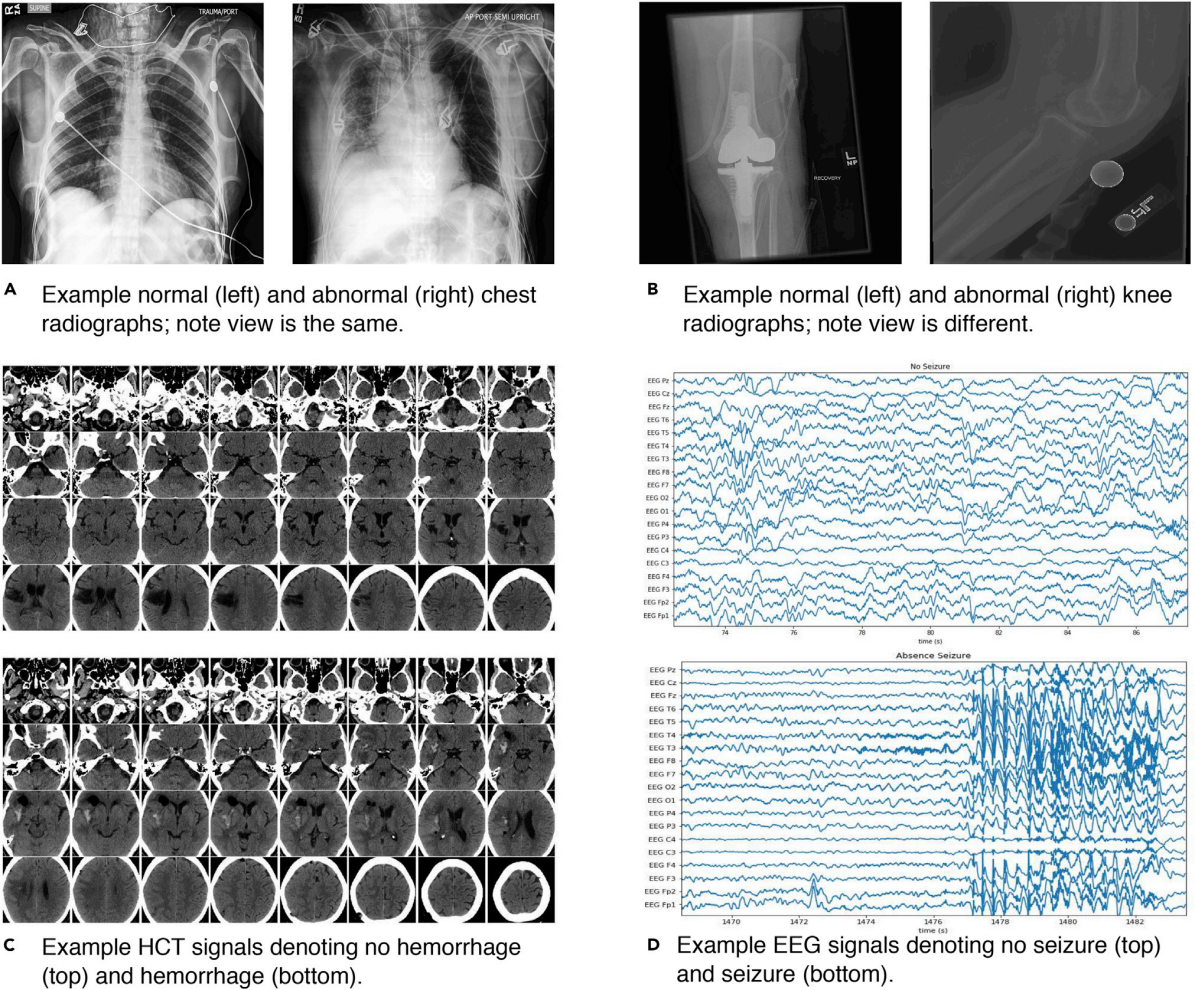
Example Target Modality Data for Four Clinical Applications Single 2D chest radiographs (A), examples of knee extremity radiographs drawn from 2D radiograph series (B), 32 slices from 3D head CT scans (HCT) with and without hemorrhage (C), and 19-channel electroencephalography (EEG) signals with and without evidence of seizure onset (D). Note that while these applications are fundamentally different in both dimensionality and modeling requirements (see Table 1), deep machine learning models supporting each can be rapidly trained using cross-modal data programming.
